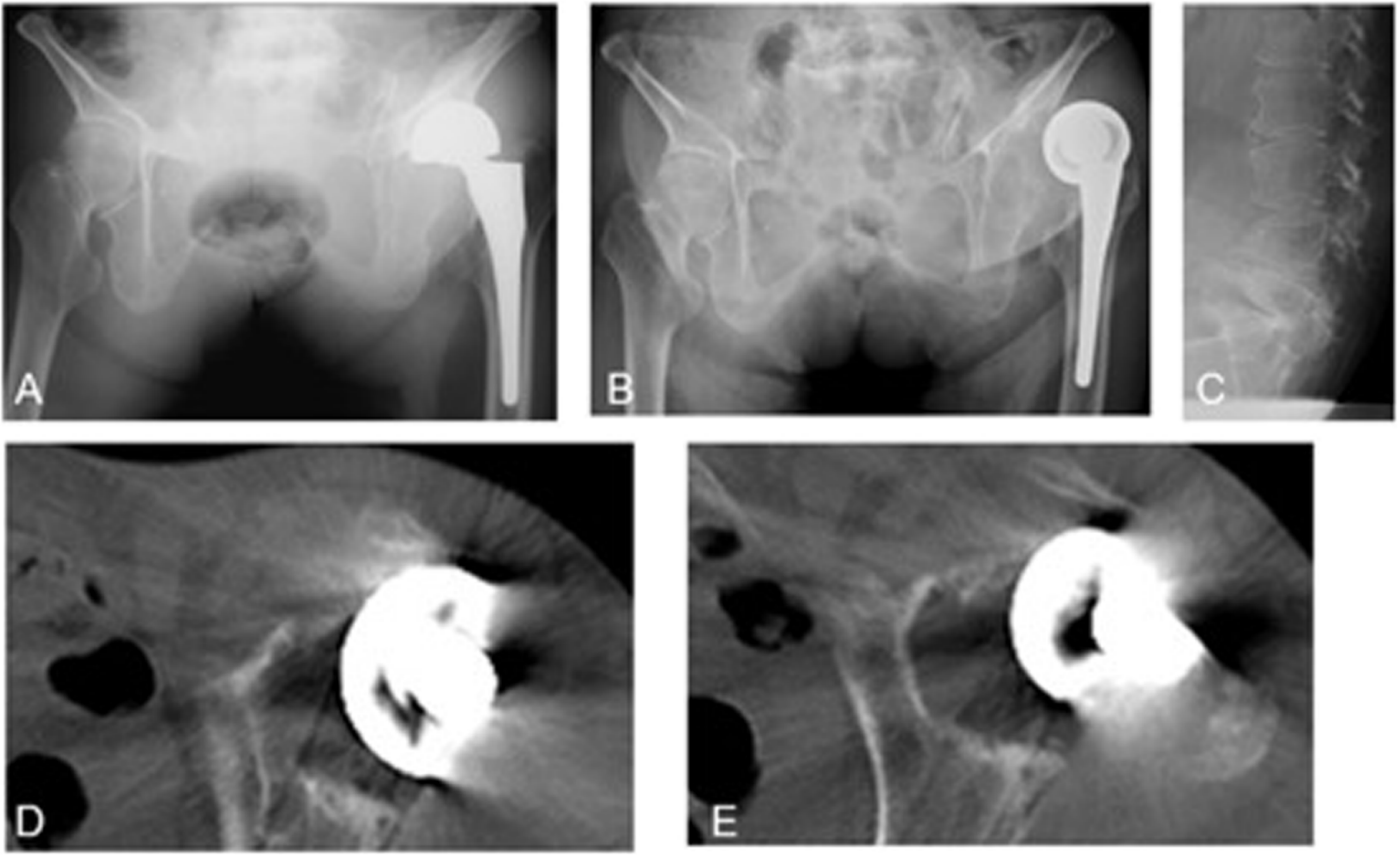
Preoperative hip X-rays and CT images. a X-ray image of the front of the hip with the patient in a standing position, taken at a previous hospital 1 year earlier, showing severe posterior pelvic tilt. b X-ray image of the front of the hip with the patient in a supine position at first visit to our hospital, showing anterior dislocation of the hemi-arthroplasty and a posterior pelvic tilt. c Lumbar sagittal X-ray image of the patient in the supine position at first visit to our hospital, showing a wedge-shaped compression fracture of the fifth lumbar vertebra and lumbar lordosis. d Preoperative axial CT images, showing a small bone fragment, which suggested an acetabulum anterior bone fracture
